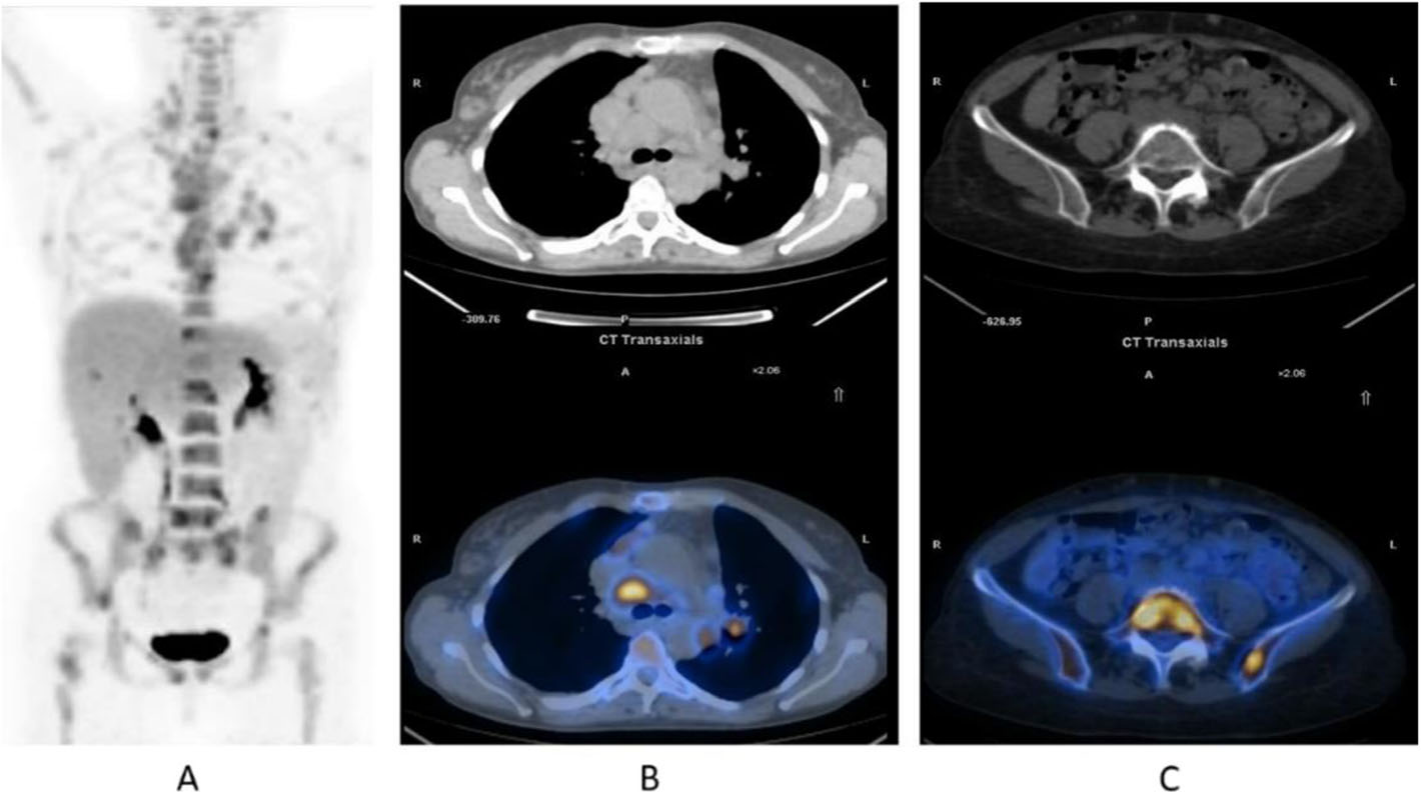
^18^F-FLT, NSCLC, before second line therapy. *Clinical history*: 57 y.o. patient with NSCLC who progressed after first line chemotherapy. *PET/CT findings*: intense tracer uptake in the left lung and in multiple large mediastinal lymph nodes and small bone lesions. a MIP. b CT and fused images of the thorax. c CT and fused images of the pelvis
